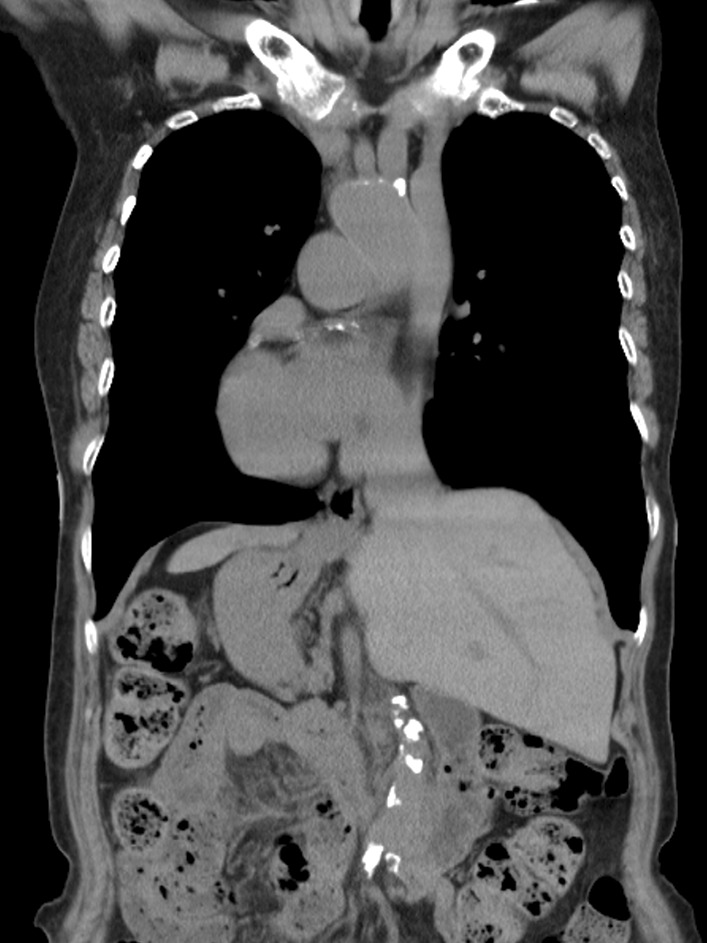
Preoperative computed tomography. All organs, including the cardiovascular system, are mirrored compared to their normal localization
